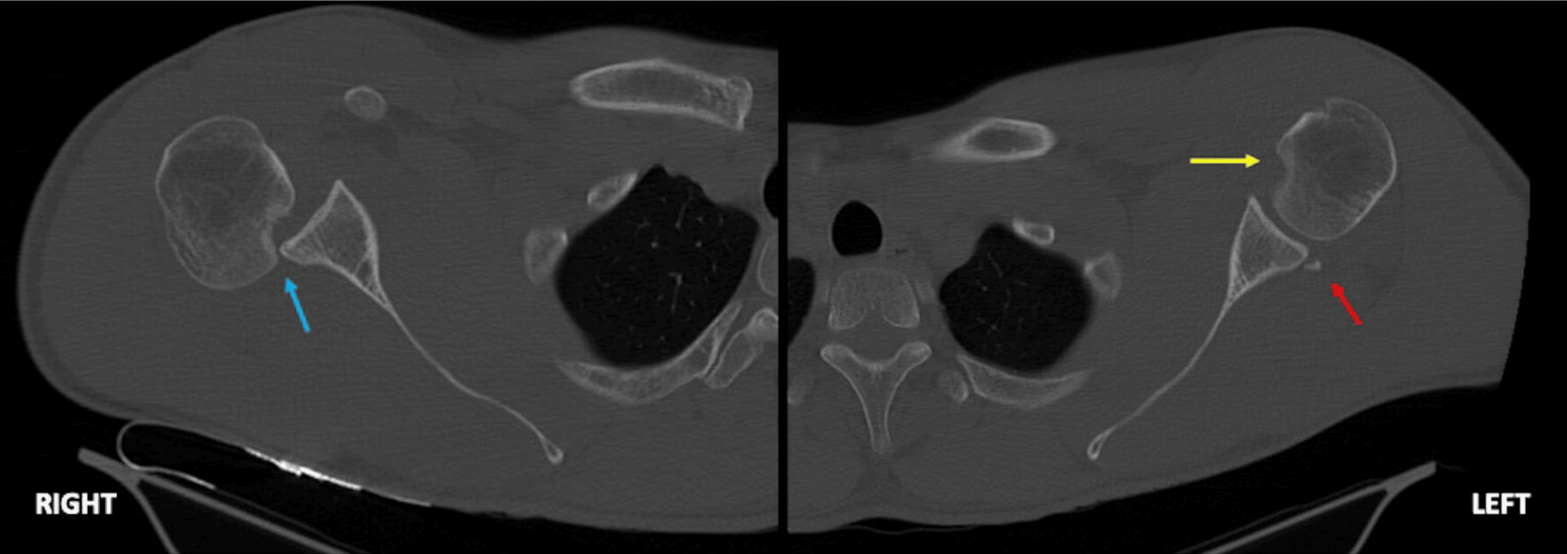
Control bilateral shoulder axial CT scan. The right shoulder scan presented a posterior shoulder dislocation with a Reverse Hill-Sachs lesion (RHSL) - highlighted by the blue arrow - and no lesions on the glenoid were noted. The left shoulder scan revealed a congruent joint with an RHSL and a Reverse Bankart bone lesion- marked by the yellow and red arrow, respectively.
